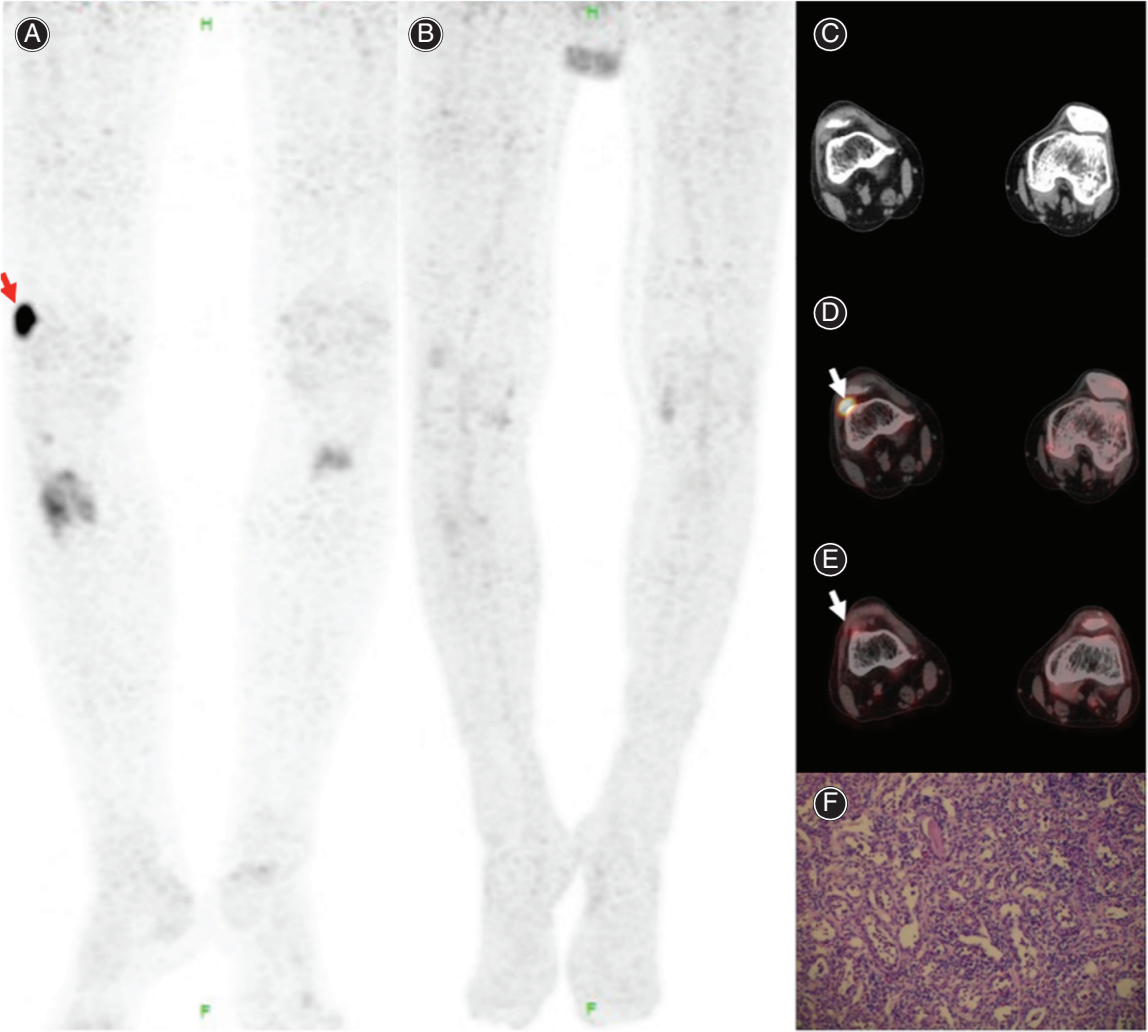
A 59‐year‐old man had difficulty working with worsening diffuse bone pain, for more than 3 years. His serum phosphate level was 0.60 mmol/L. ^68^Ga DOTATATE PET/CT image (A, C) demonstrated an intense activity with maximum standardized uptake value (SUV_max_) of 17.2 (arrow) behind the right patella (C, white arrow), whereas ^18^F‐FDG PET/CT image (B, E) revealed no increased uptake in the soft tissue in the same lesion. The finding was suggestive of a causative tumor. After surgically removing a tumor 1.2 cm in largest dimension from the right knee, the patient's symptoms promptly improved, and his postsurgical serum level returned to normal at 1.09 mmol/L. The results of the pathological examination confirmed the phosphaturic mesenchymal tumor (F).
